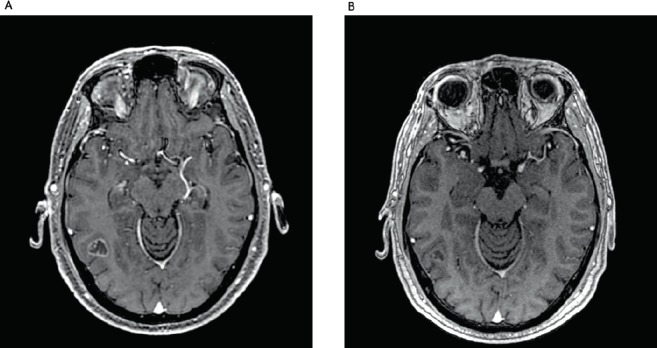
(A): Brain MRI demonstrating right occipitotemporal lesion measuring 1.8 cm × 1.4 cm. (B): Two months after rechallenge of osimertinib and reduction of the lesion, measuring 0.8 cm × 0.5 cm.
